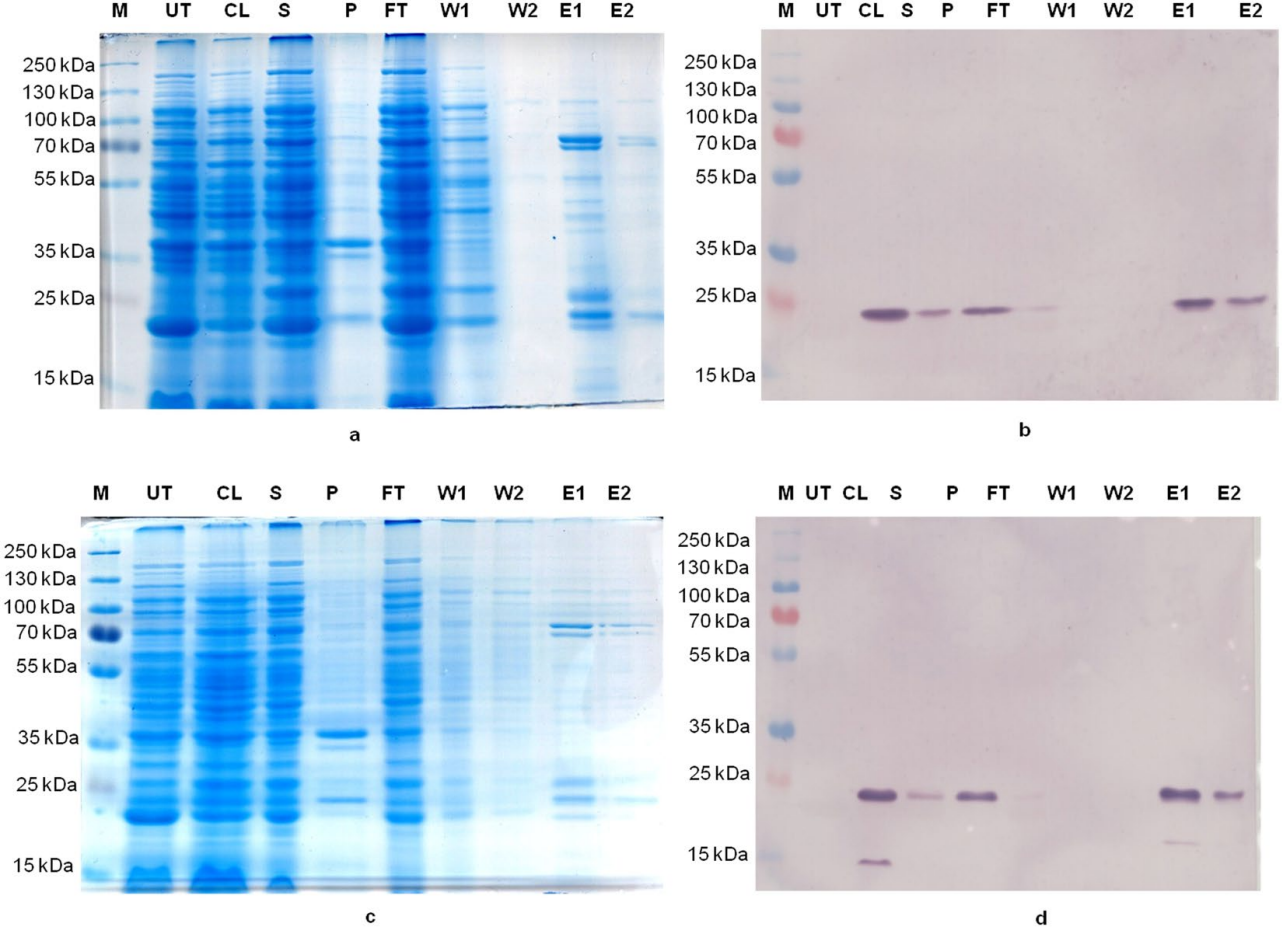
Small scale protein purification of LusPLA_2_s by Ni-NTA purification column by affinity chromatography. (a) SDS-PAGE analysis of the protein fractions of pET301/CT-DEST harbouring LusPLA_2_I-6xHis fusion protein. (b) Detection LusPLA_2_I-6xHis fusion protein by western blotting using anti-His antibodies. (c) SDS-PAGE analysis of the protein fractions of pET301/CT-DEST harbouring LusPLA_2_II-6xHis fusion protein. (d) Detection of LusPLA_2_II-6xHis fusion protein by western blotting using anti-His antibodies. M - Pageruler plus prestained protein ladder, UT-Untransformed BL21-RIPL, CL-crude lysate of transformed BL21-RIPL, S-supernatent, P-pellet, FT-flow through, W1-first wash, W2- second wash, E1- first elute and E2- second elute.
